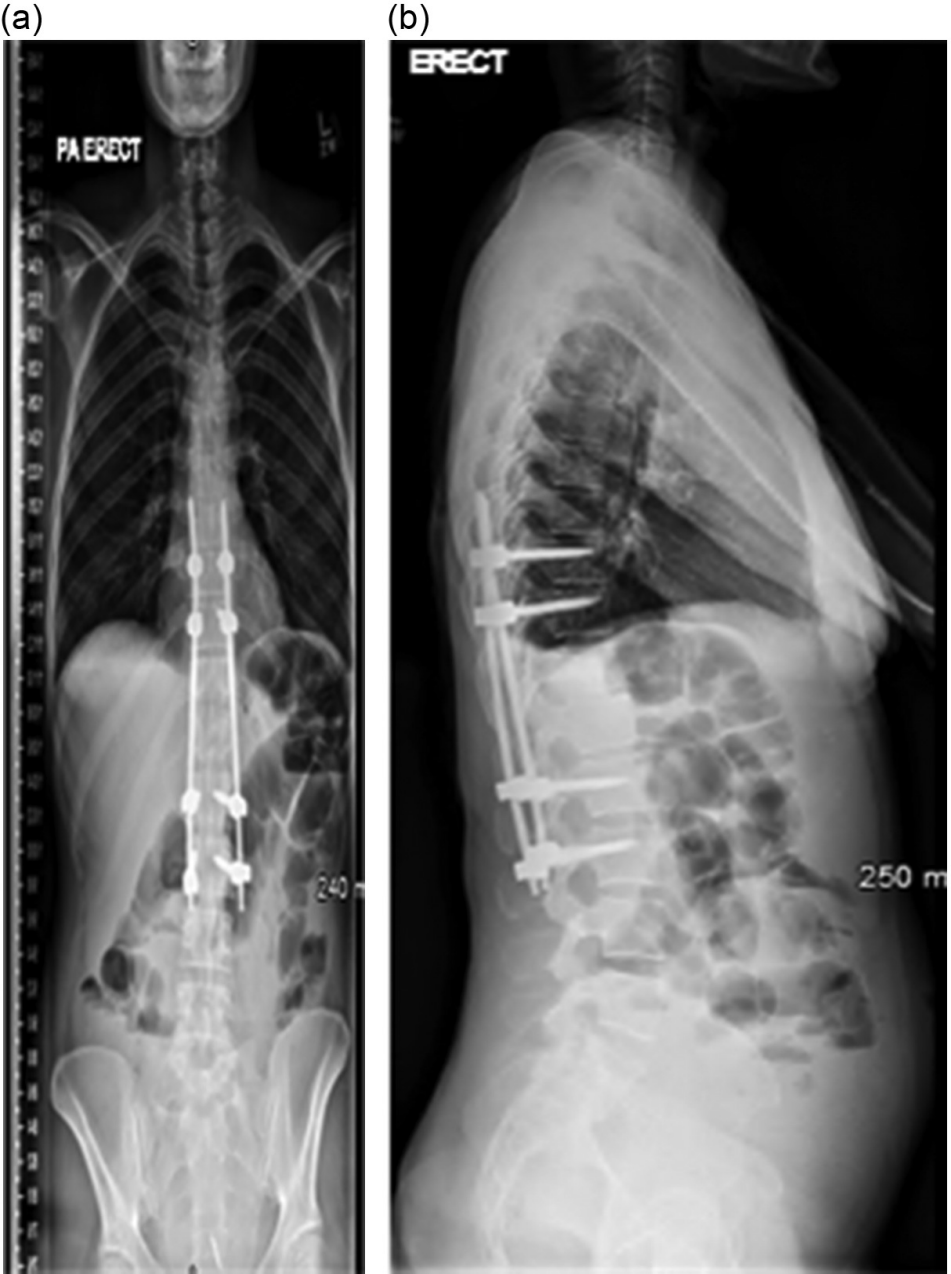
(a) Anteroposterior radiograph of the whole spine. (b) lateral radiograph of the whole spine. Stabilisation with screws and rods can clearly be seen from T10 to L3.
